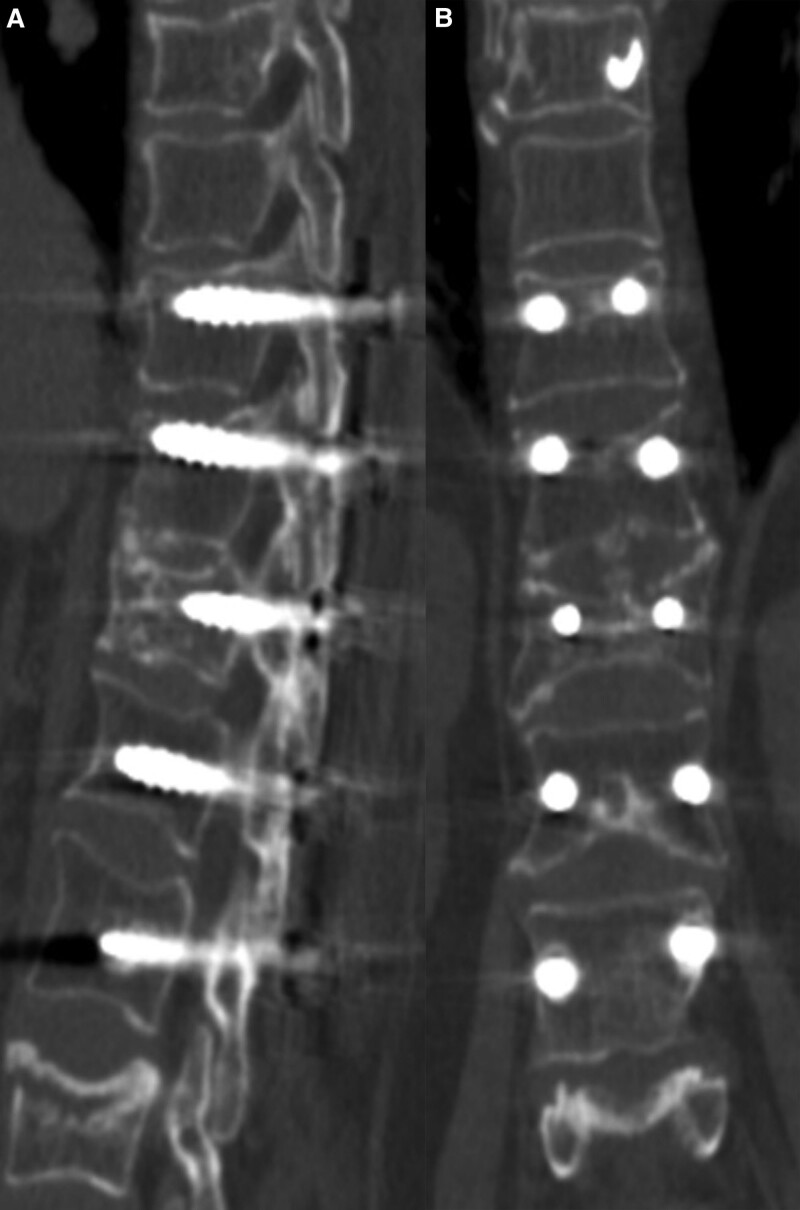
Good vertebral height, bone healing and new bone formation in T12 vertebral body. A, B: Axial (A) and coronal (B) CT scans in T12 vertebral body during the 36 months of postoperative follow-up. CT = computer tomography.
